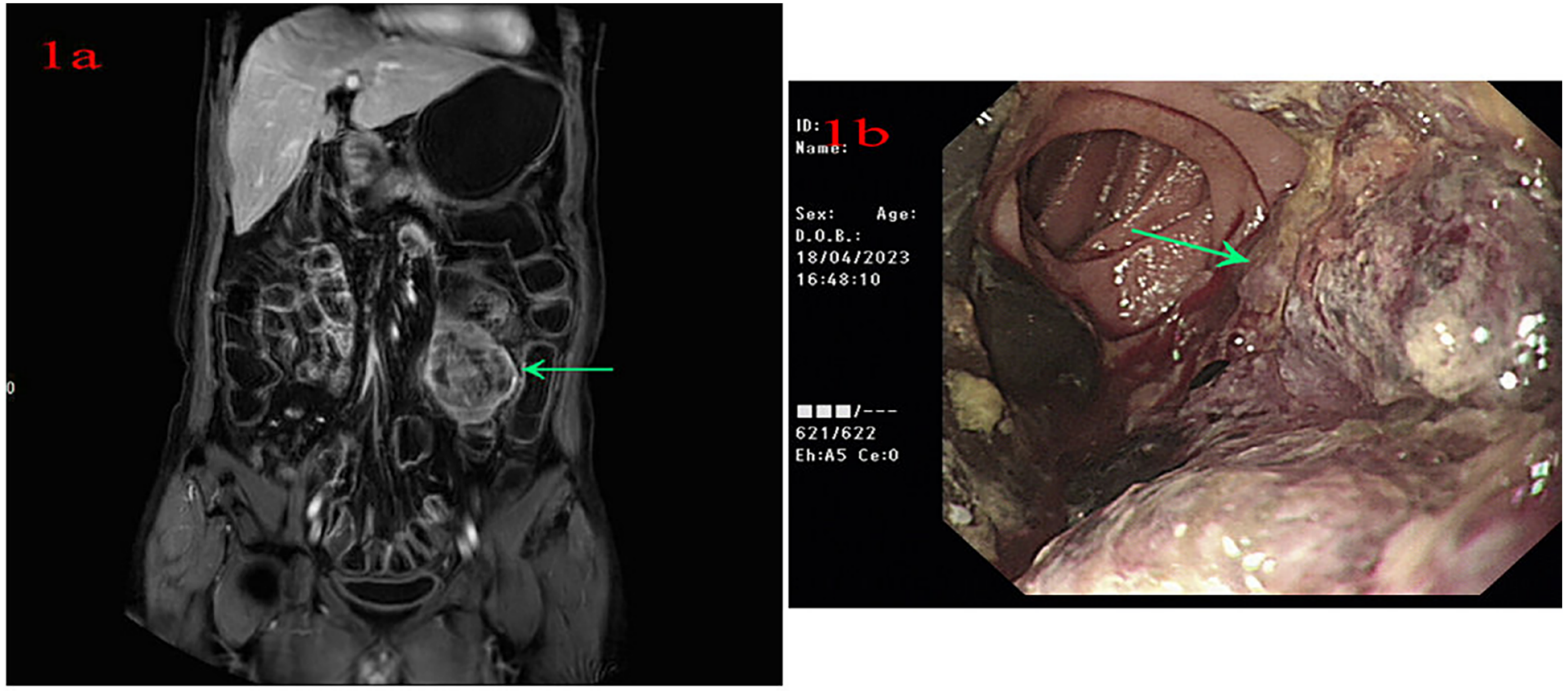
(a) Abdominal magnetic resonance imaging indicates a left mid-abdominal mass. (b) Small bowel enteroscopy shows infiltrating lesions of periannular ulcer at the jejunum.
